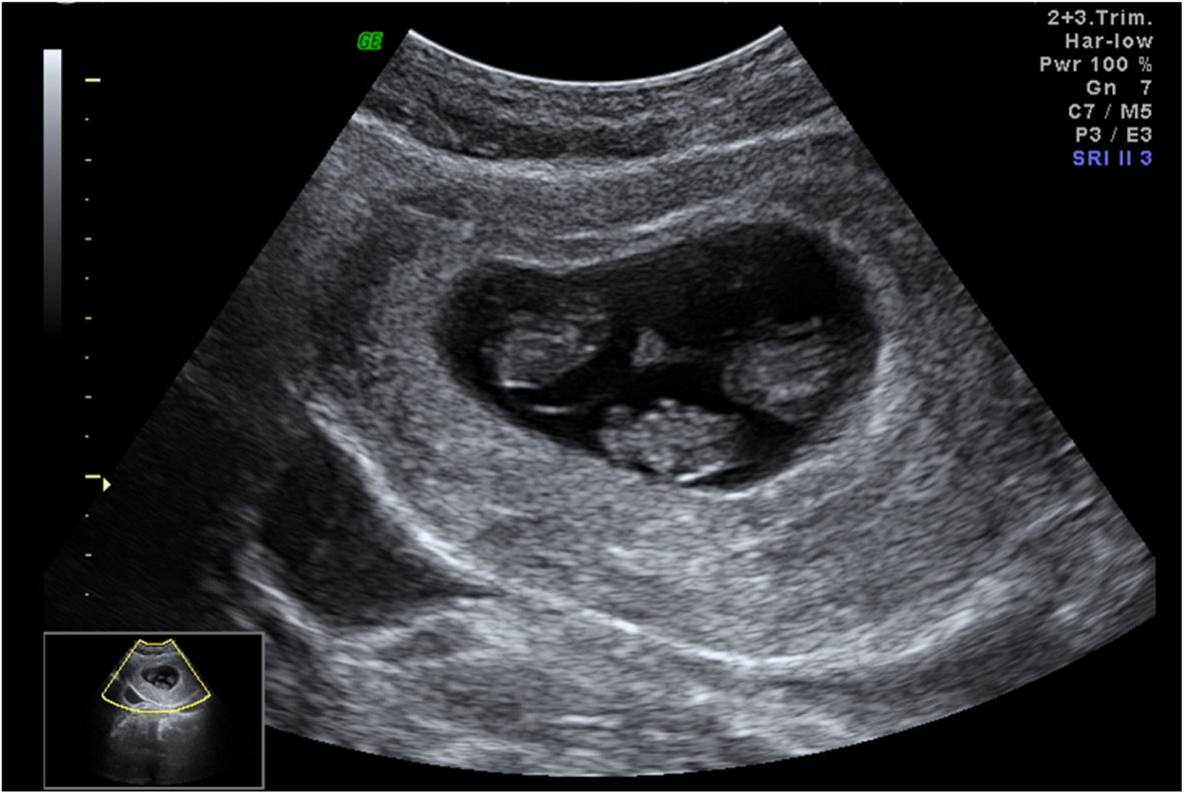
Prenatal transabdominal ultrasound at 8 weeks of gestation demonstrated three viable fetuses with one shared placenta and three amniotic cavities
